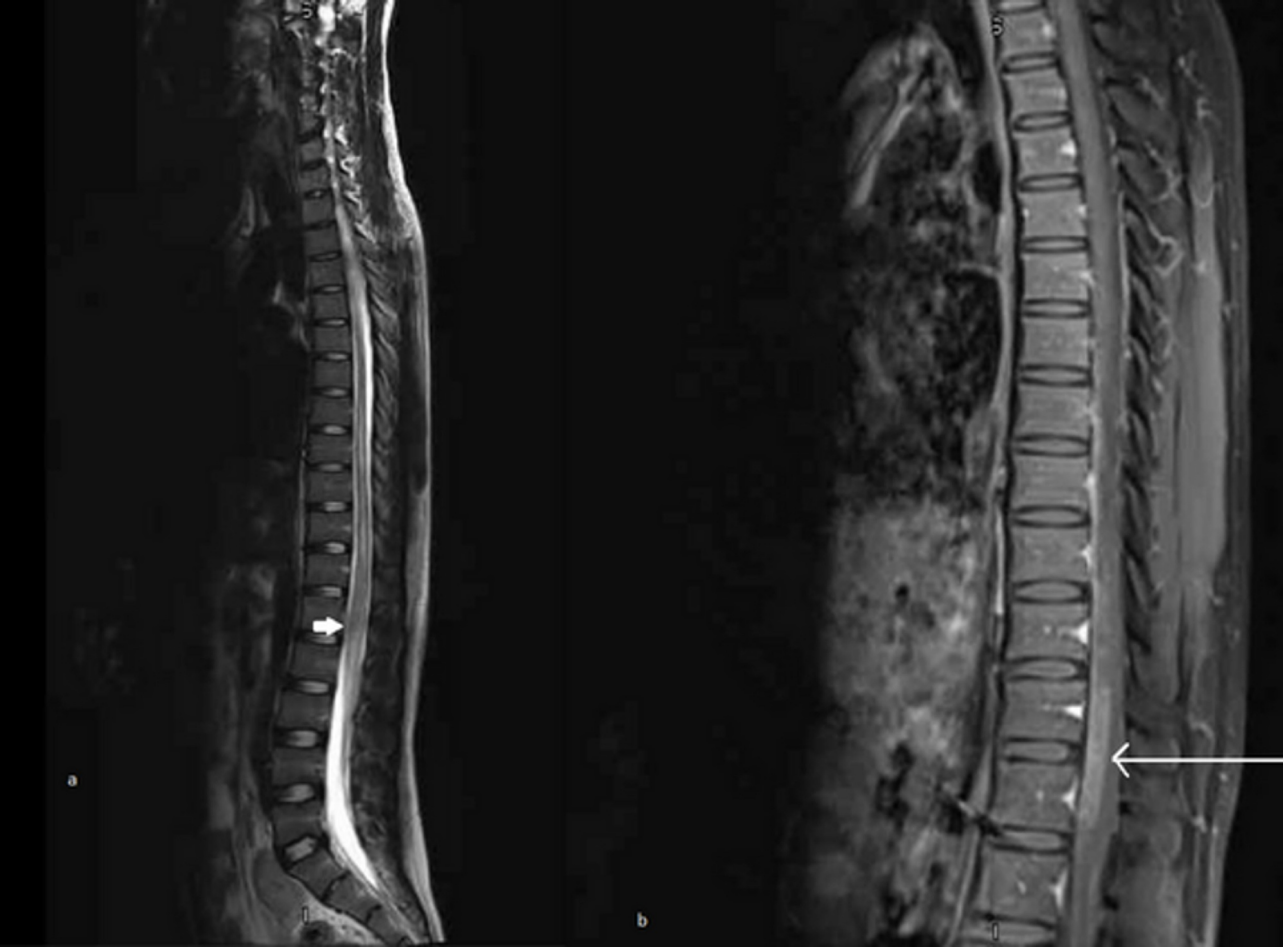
MRI thoracolumbar spine with contrast showing interval increase in intramedullary T2 enhanced signals. There is also a prominent enhancement of cauda equina root. Oedema of conus (arrow in image a). New patchy enhancement noted in the spinal cord at T10-T11 level (image b)
